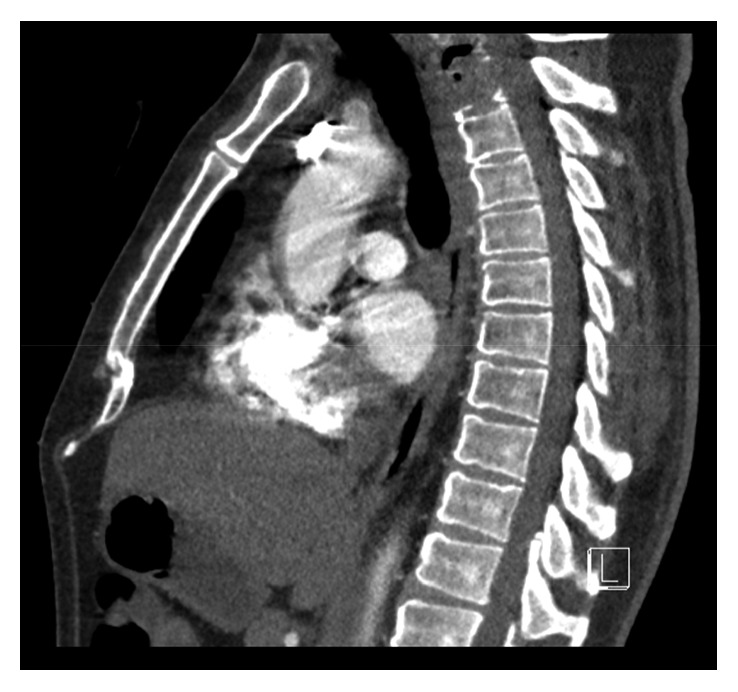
CT spine demonstrating local invasion of the tumor and air within the vertebral bodies.
